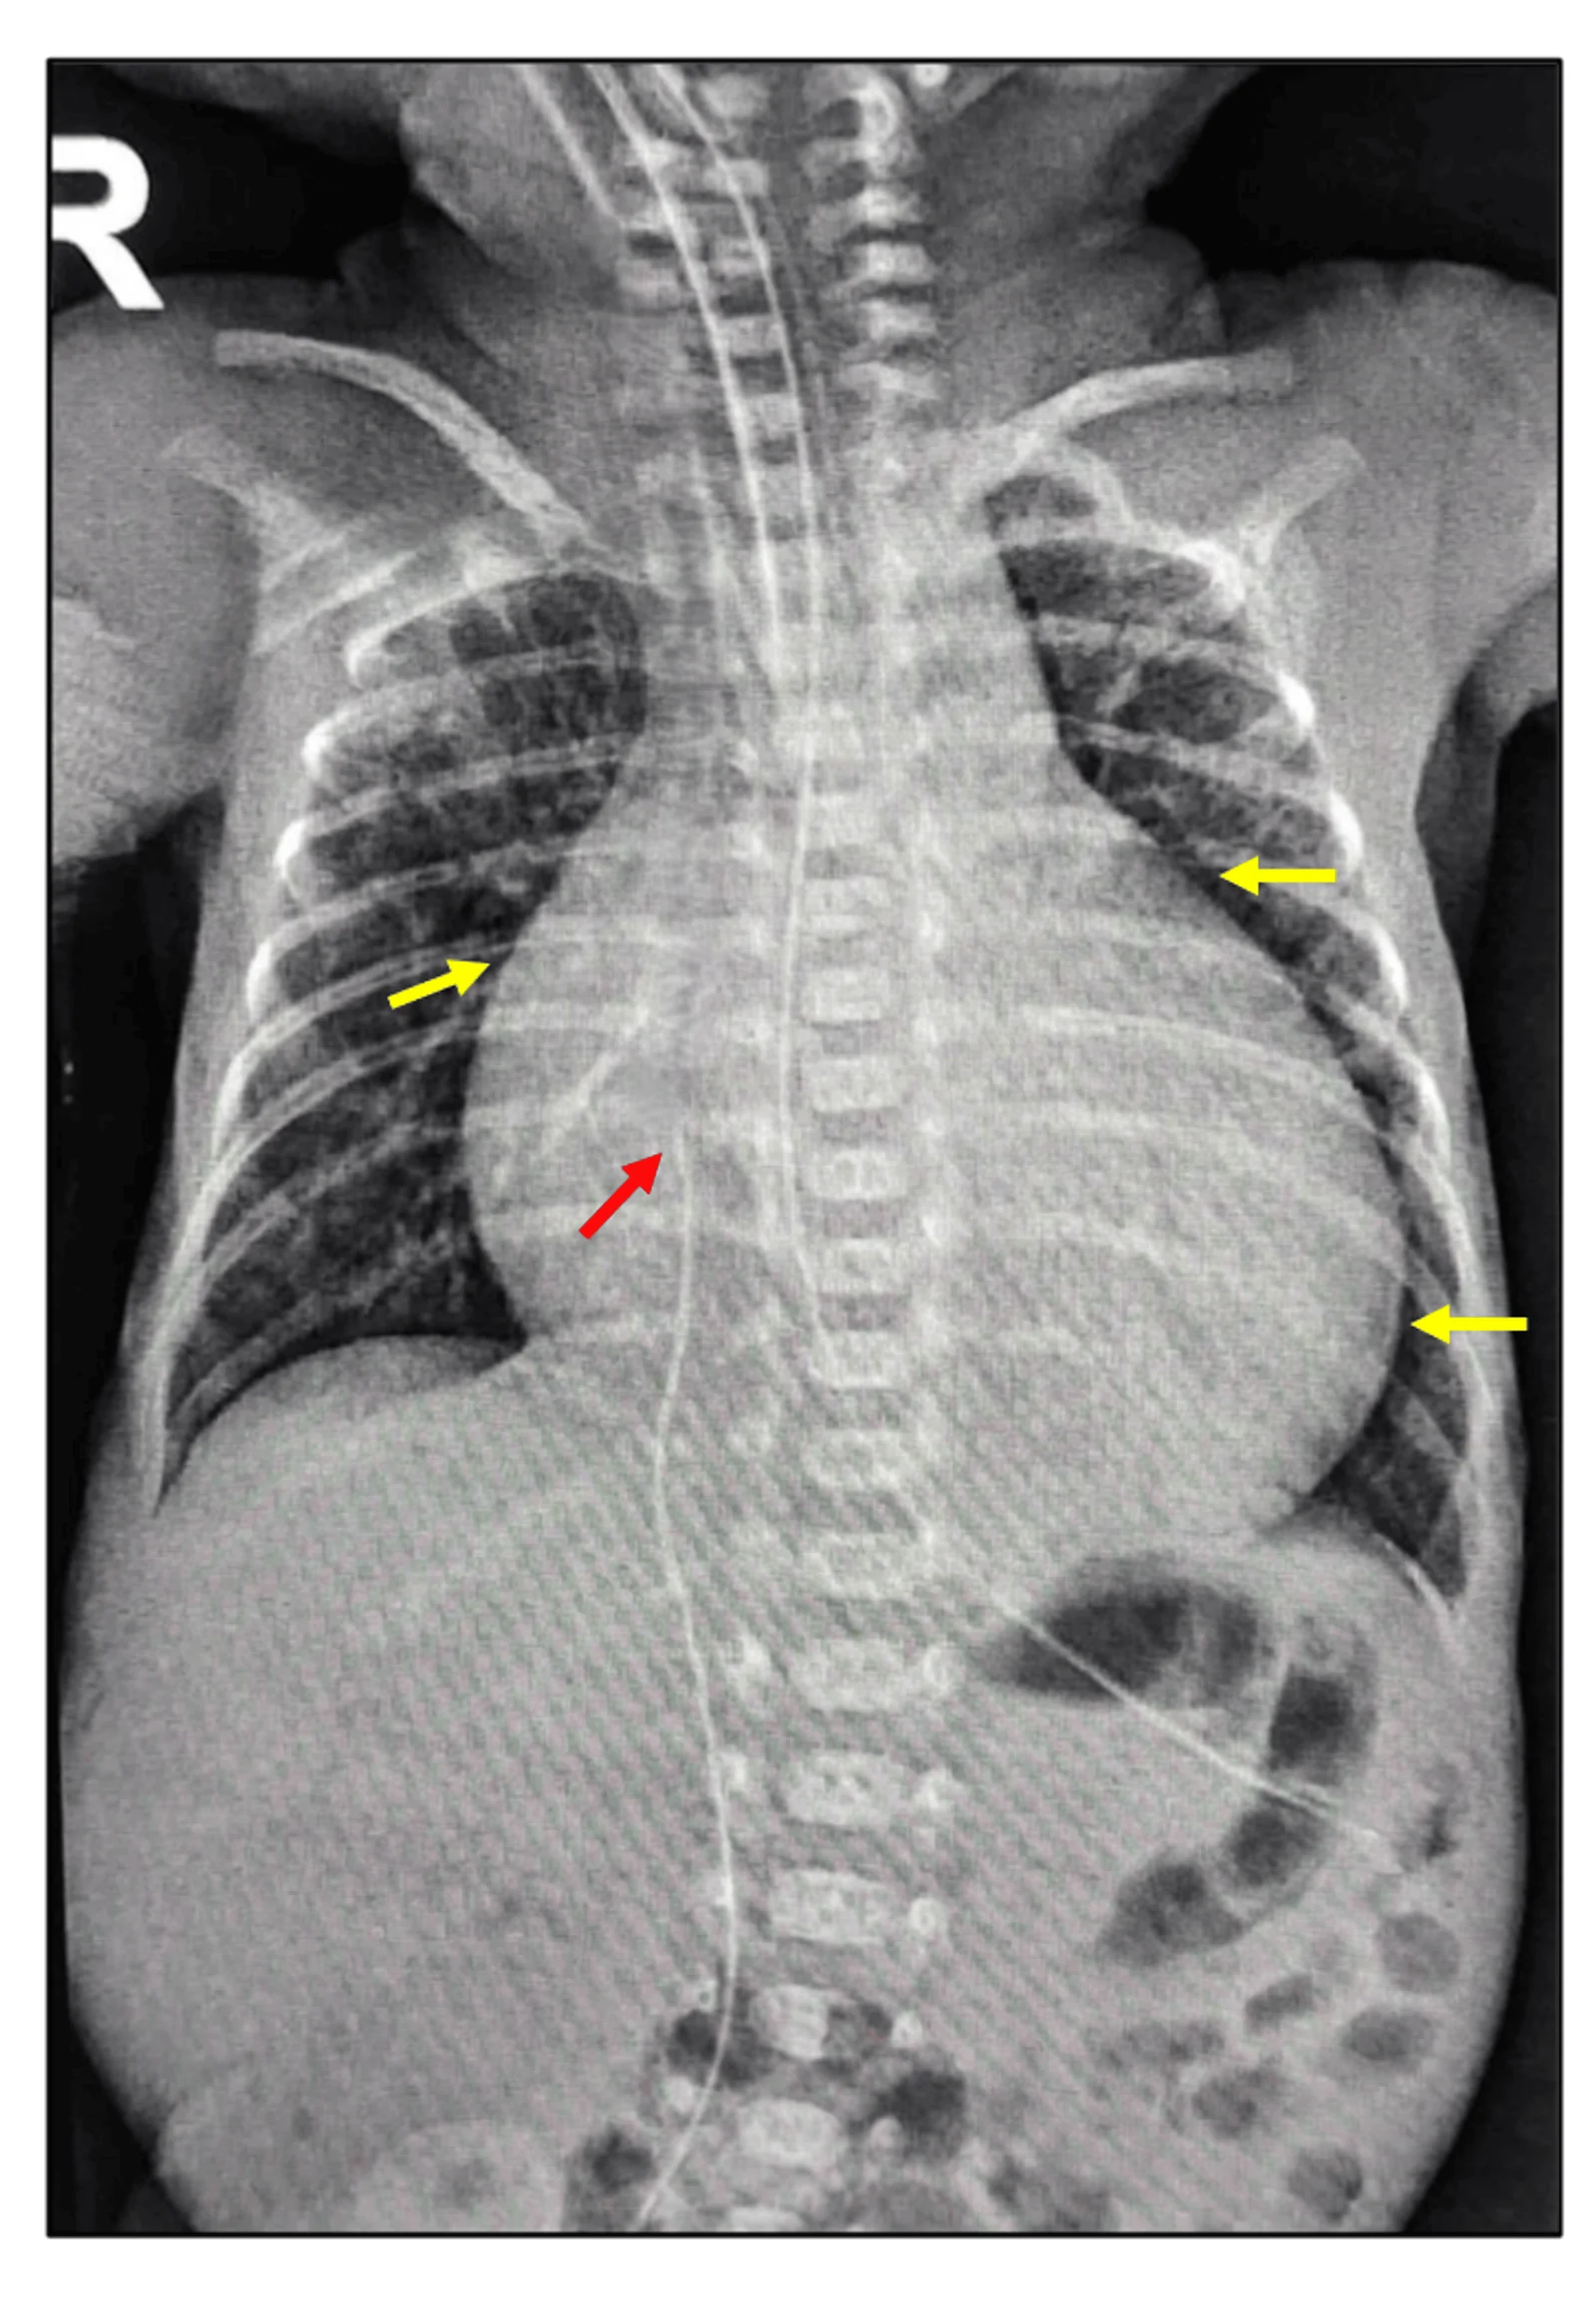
Anteroposterior chest radiograph demonstrating marked cardiomegaly with a characteristic "water-bottle" cardiac silhouette, highly suggestive of a massive pericardial effusion (yellow arrows). The UVC tip projects at the T9 vertebral level, below the diaphragm, indicating a low-lying catheter position (red arrow). UVC: Umbilical venous catheter
